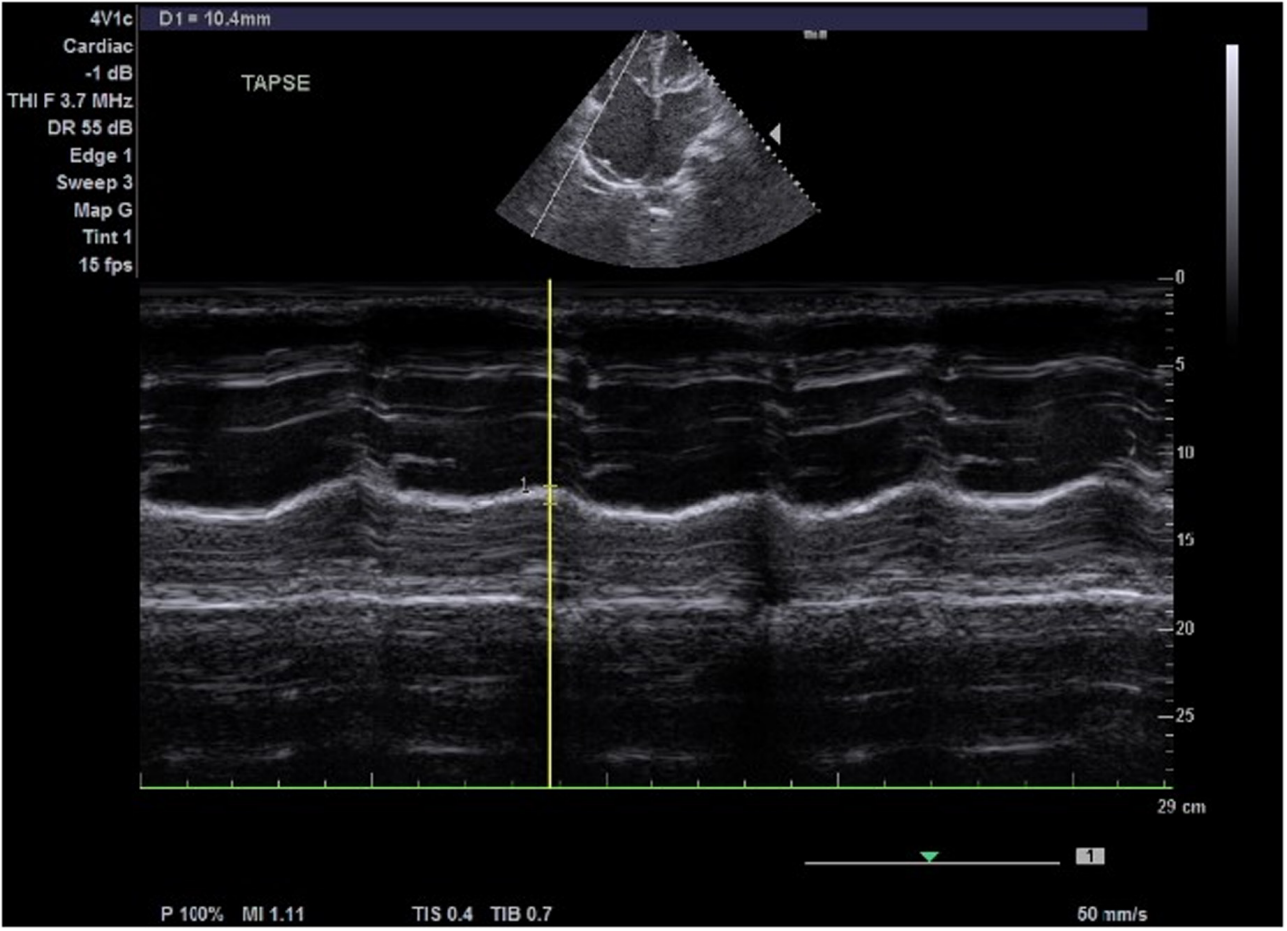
M‐mode echocardiogram showing tricuspid annular plane systolic excursion of 10.4 mm.
